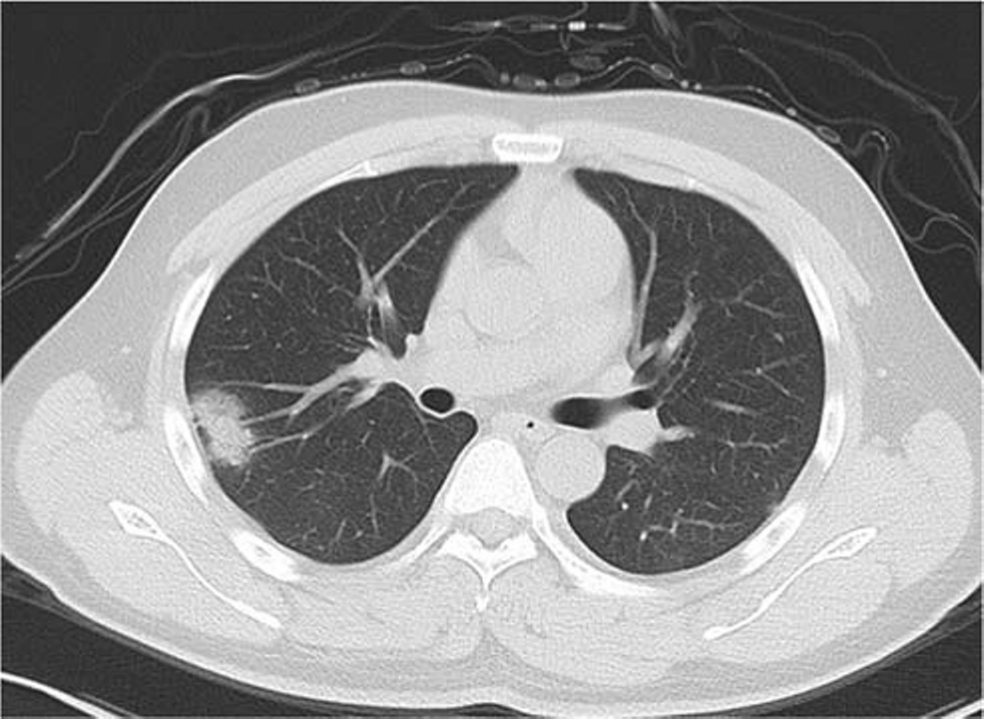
A 23-year-old female with a travel history to Wuhan presenting with fever. Axial noncontrast CT image shows a consolidation with ground-glass opacities in the peripheral region by the right upper lobe. Air bronchogram is found in lesion. The maximum diameter of lesion is 2.8 cm. The right upper lobe score is 1 because of the involved lung parenchyma less than 1/4
